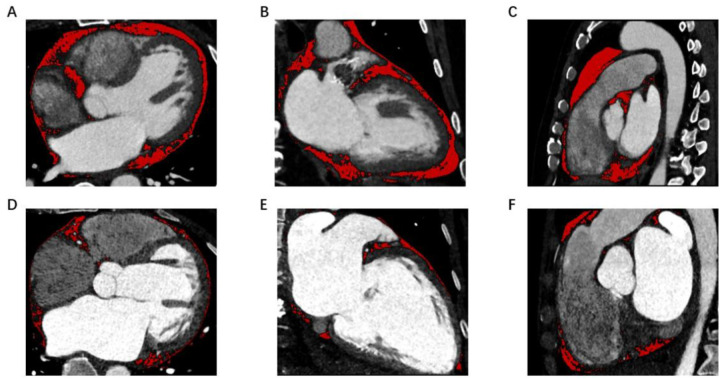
Measurement of EAT volume in different type of AF with heart failure. EAT volume was larger in PsAF (A–C) than PaAF (D–F) in patients with Heart Failure. The EAT volume was analyzed using CT image data.
